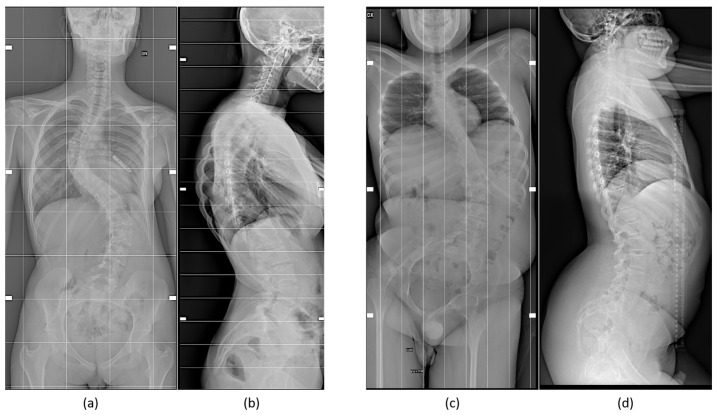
Female patient (a,b), 15 years old, with a severe thoraco-lumbar scoliosis, X-rays AP (a) and lateral (b) views. Idiopathic scoliosis has a known higher incidence rate in female patients. Male patient (c,d), 16 years old, with spinal muscular atrophy, X-rays AP (c) and lateral (d) views showing a severe lumbar scoliosis. Male patients usually have a lower incidence rate of scoliosis compared to female counterparts, apart from syndromic patients, but higher severity.
